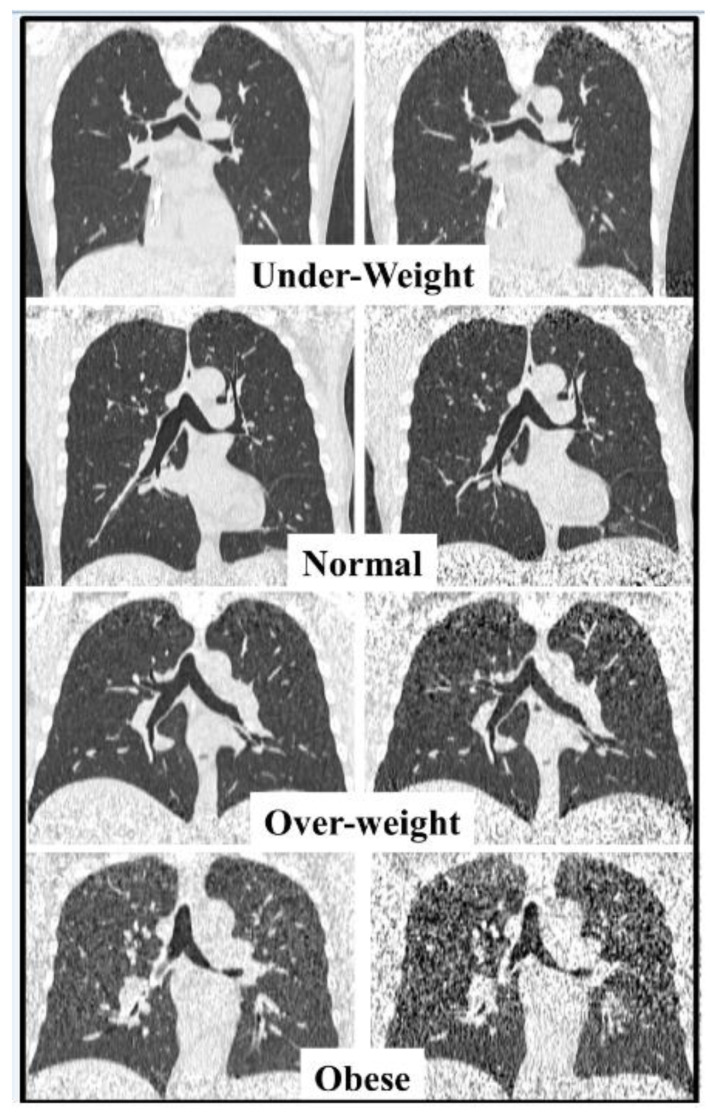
Four paired coronal CT images in patients scanned with LDCT protocol (left column) and ultra-LDCT protocol (right column). The ultra-LDCT image in the obese patient degrades substantially especially in the lung apex and close to the diaphragm due to photon starvation.
